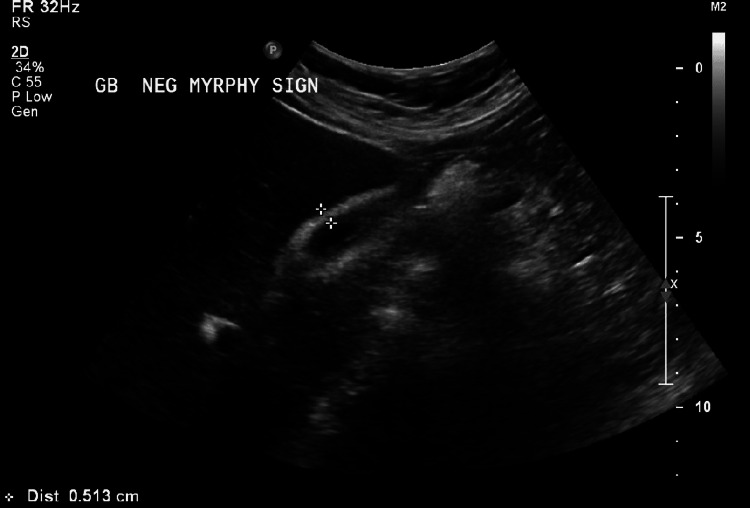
Gallbladder wall thickness of 5 mm with no gallstones noted on ultrasound.
